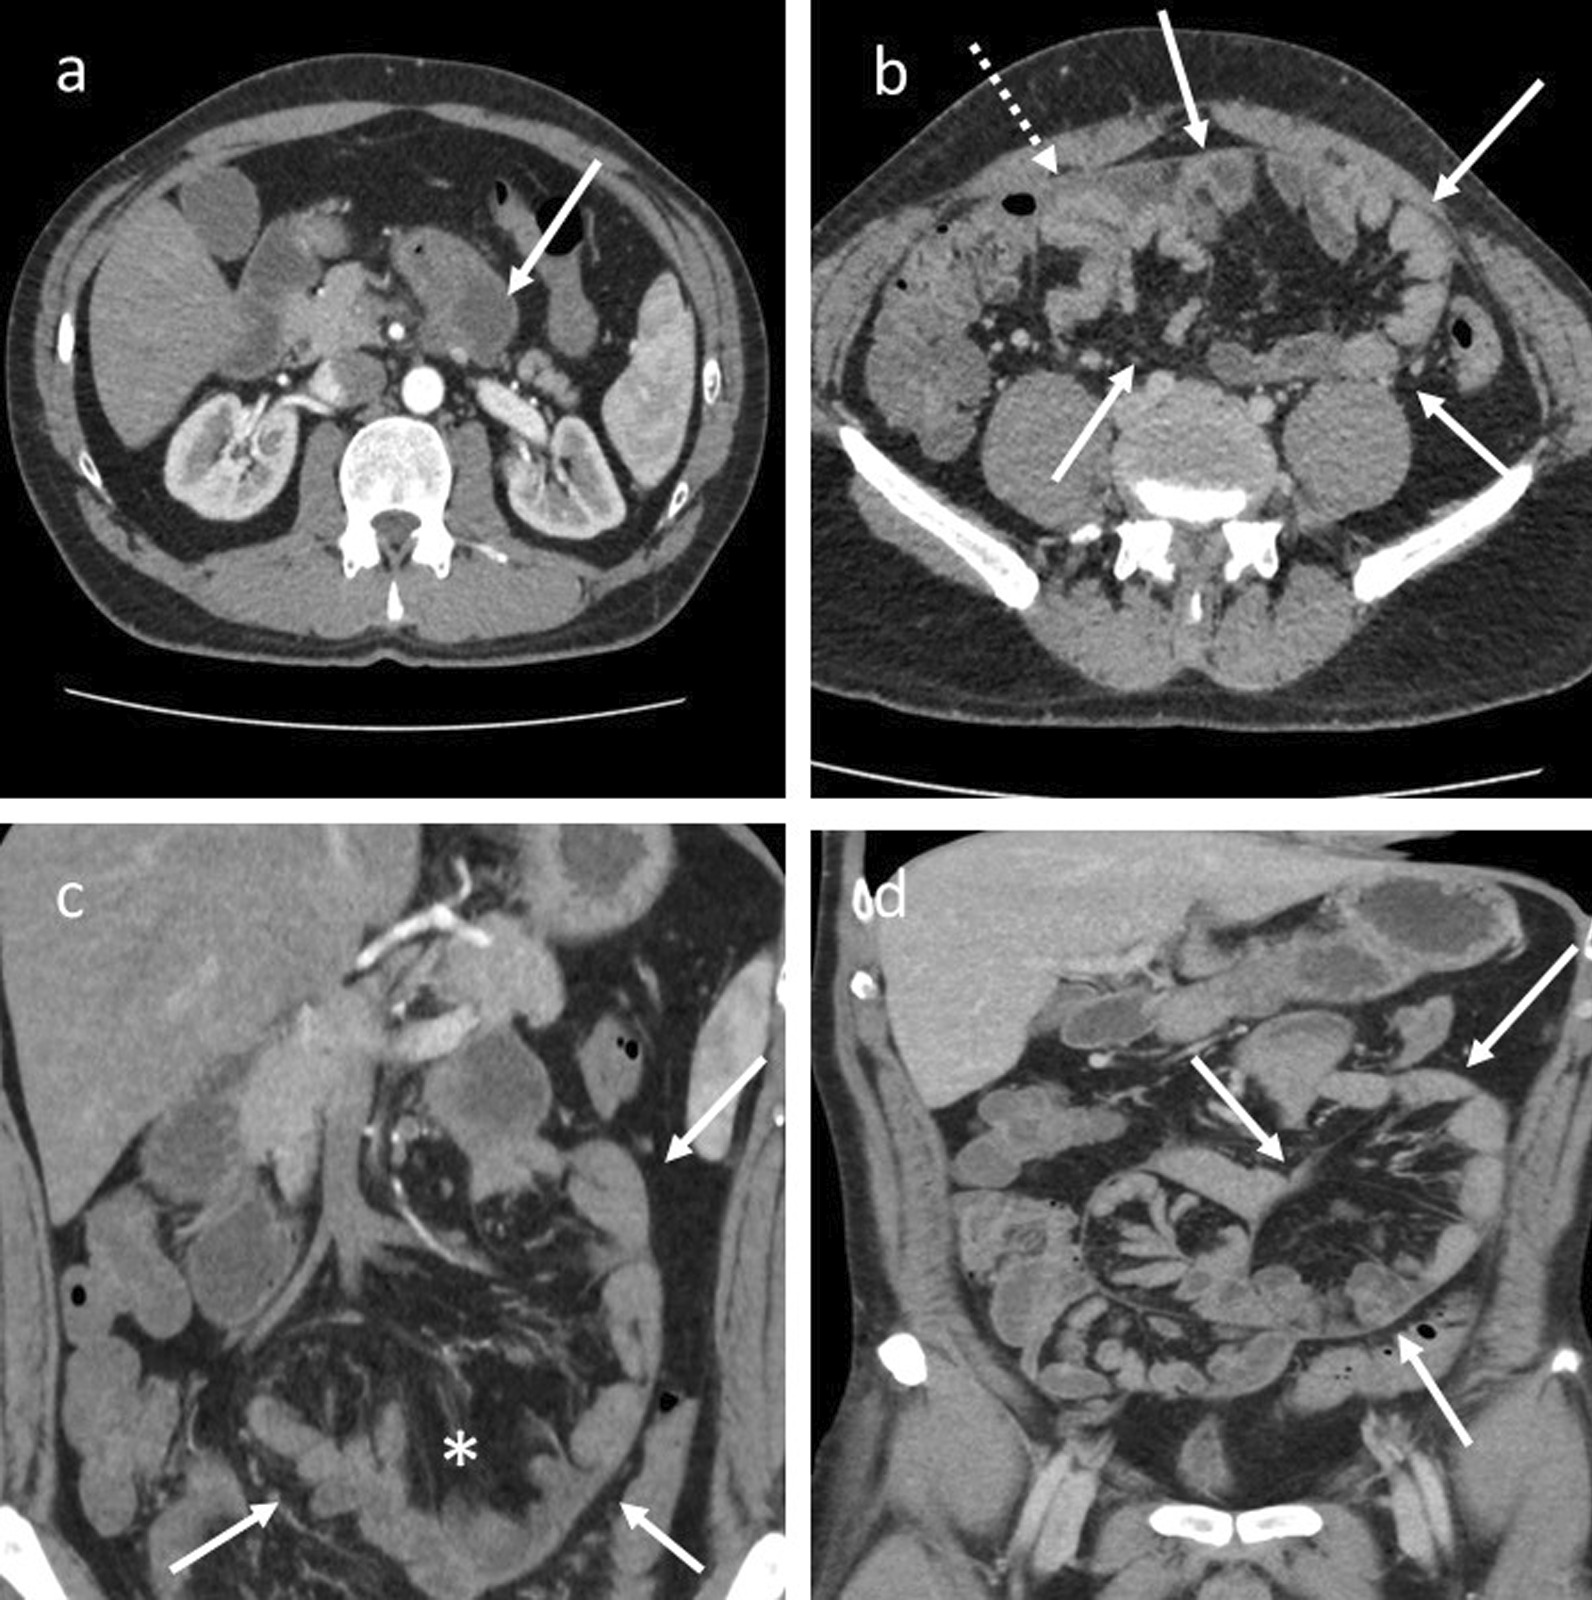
Contrast-enhanced computed tomography (a–d). a Axial cross-section demonstrating dilated proximal bowel loops (arrow). b–d Axial cross-section and coronal-cross section demonstrating “sac-like” configuration of small bowel loops (arrows), free fluid (dashed arrow), and mesenteric fat stranding (asterisk)
